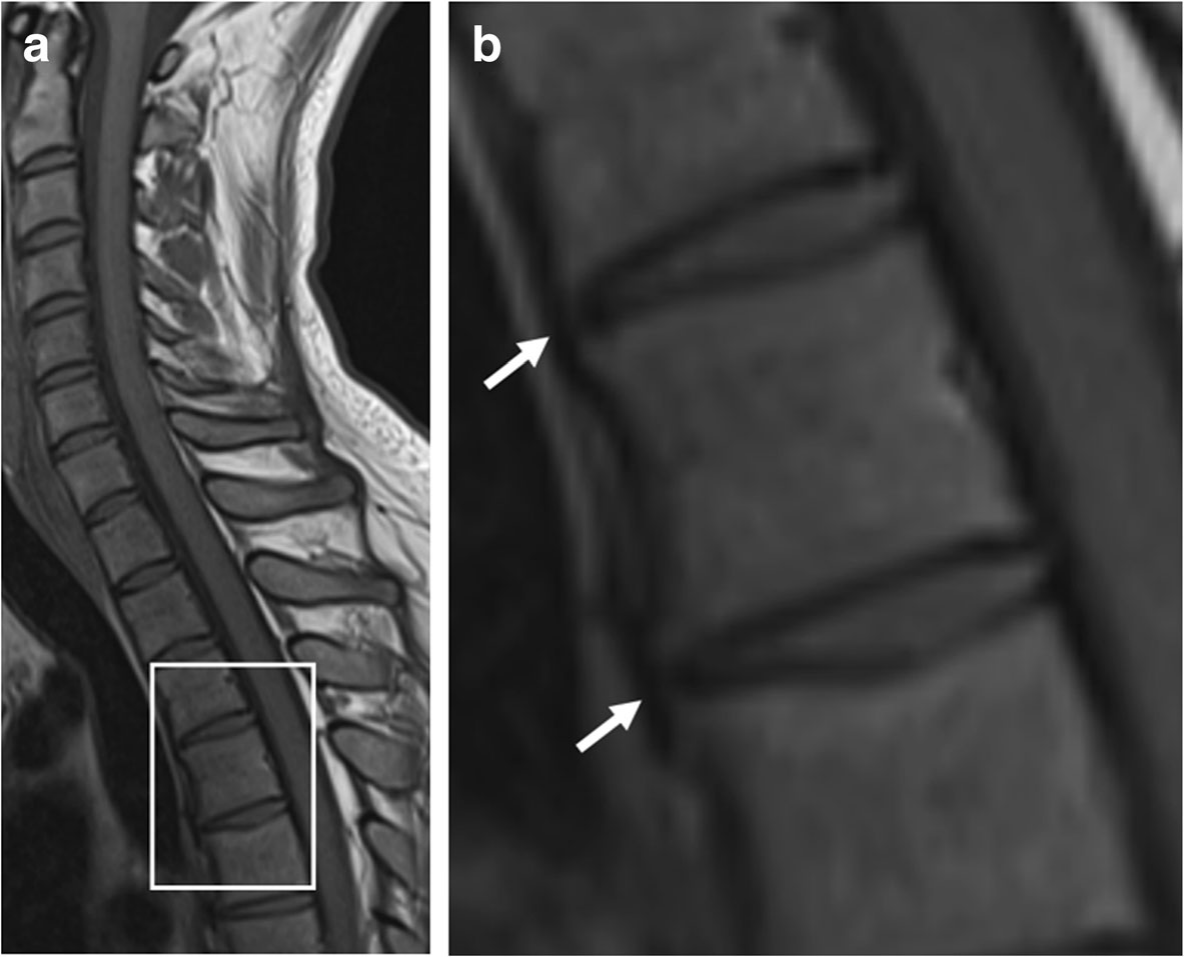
Non-bridging syndesmophytes. Sagittal T1-weighted MR image shows anterior syndesmophytes (arrows) in a patient without SpA. No other disco-vertebral units besides Th3-Th4 and Th4-Th5 show bony changes. Note: b is an enlargement of the region outlined in a
